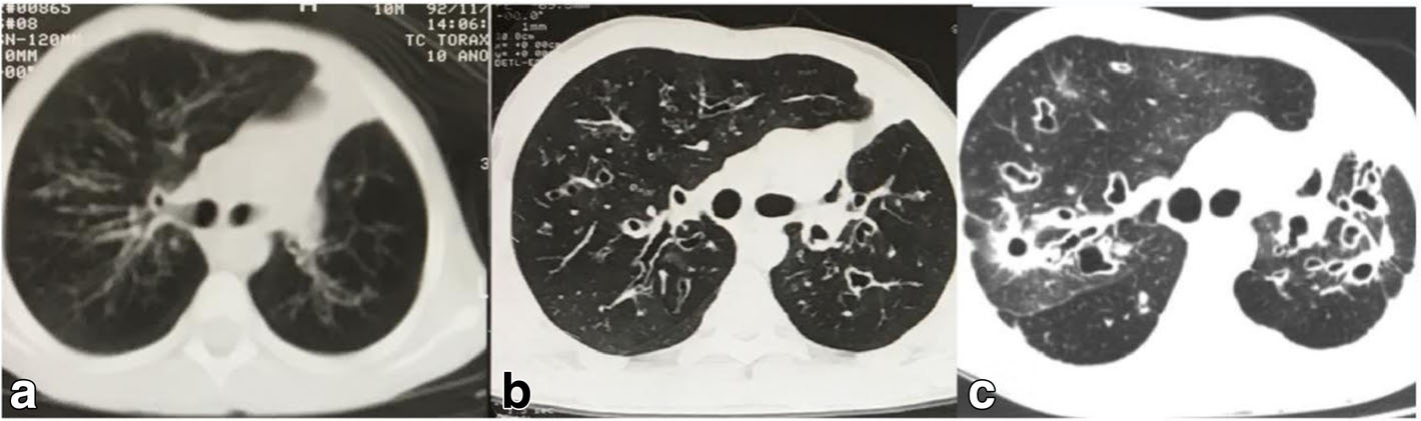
High resolution computed tomography images: 1992 (a); 2000 (b); 2015 (c)
